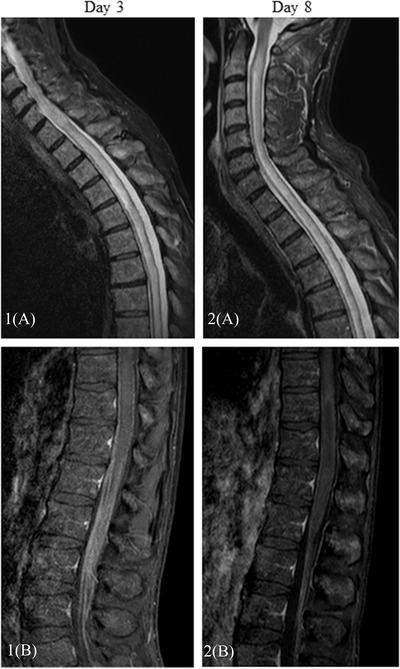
(1A) D3 magnetic resonance imaging (MRI)/sagittal short tau inversion recovery (STIR) images: extensive T2 hypersignal of the medulla, tumor‐like appearance. (1B) D3 MRI/sagittal T1 fat‐saturated post‐contrast images: poorly delineated thoracic medulla contrast patches. Clear contrast of the roots of the cauda equina. (2A) D8 MRI/sagittal STIR images: decrease of the tumescent aspect of the medulla. Stability of the T2 hypersignal extent of the medulla. (2B) D8 MRI/sagittal T1 fat saturated post‐contrast images: significant decrease in medullary contrast, especially in the roots of the cauda equina
